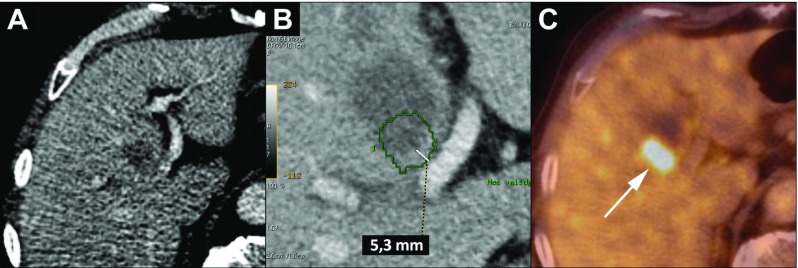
An 83-year-old man with a metastasis of a colon carcinoma and incomplete tumor coverage after ablation. a The index tumor is situated adjacent to the left portal vein. b Registration of the segmentation of the index tumor on the 24 h post-ablation ce-CT scan is projected 5.3 mm outside the boundaries of the ablation zone (Category C). c PET/CT fusion image at 8 weeks shows a LTP in this region (arrow). This LTP was successfully reablated, with follow-up of 7 years and 2 months
